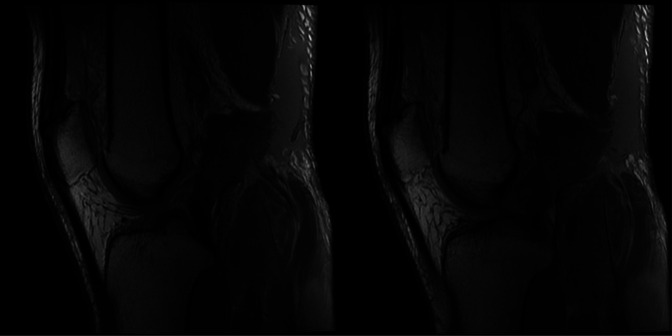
T1-weighted MRI of the right knee demonstrating complete rupture of anterior cruciate ligament and posterior cruciate ligament with associated gastrocnemius avulsion, indicative of an unstable tibiofemoral knee dislocation. MRI images obtained after the placement of knee-spanning external fixation.
